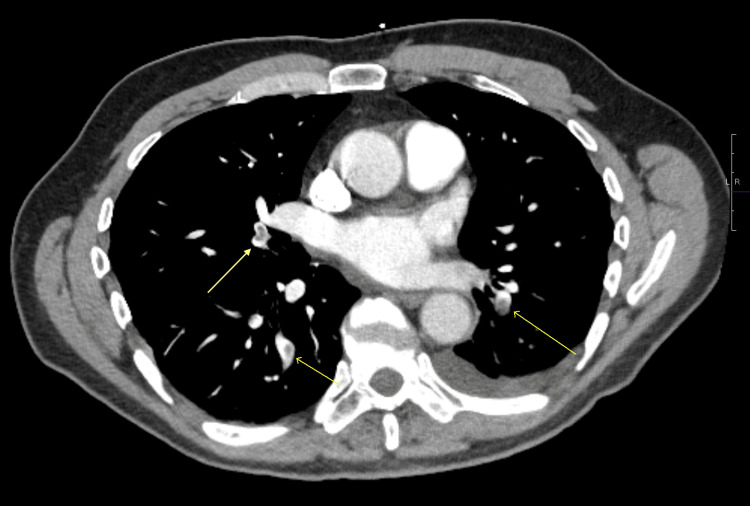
CTPA showing bilateral pulmonary emboli CTPA: CT pulmonary angiography
